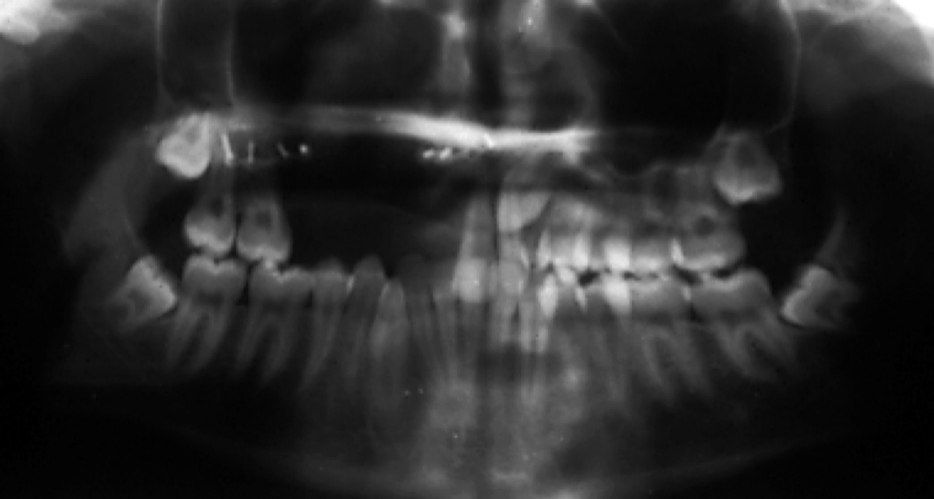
Case report 2. Panoramic X-ray after bone grafts from iliac crests fixed with titanium plates.
